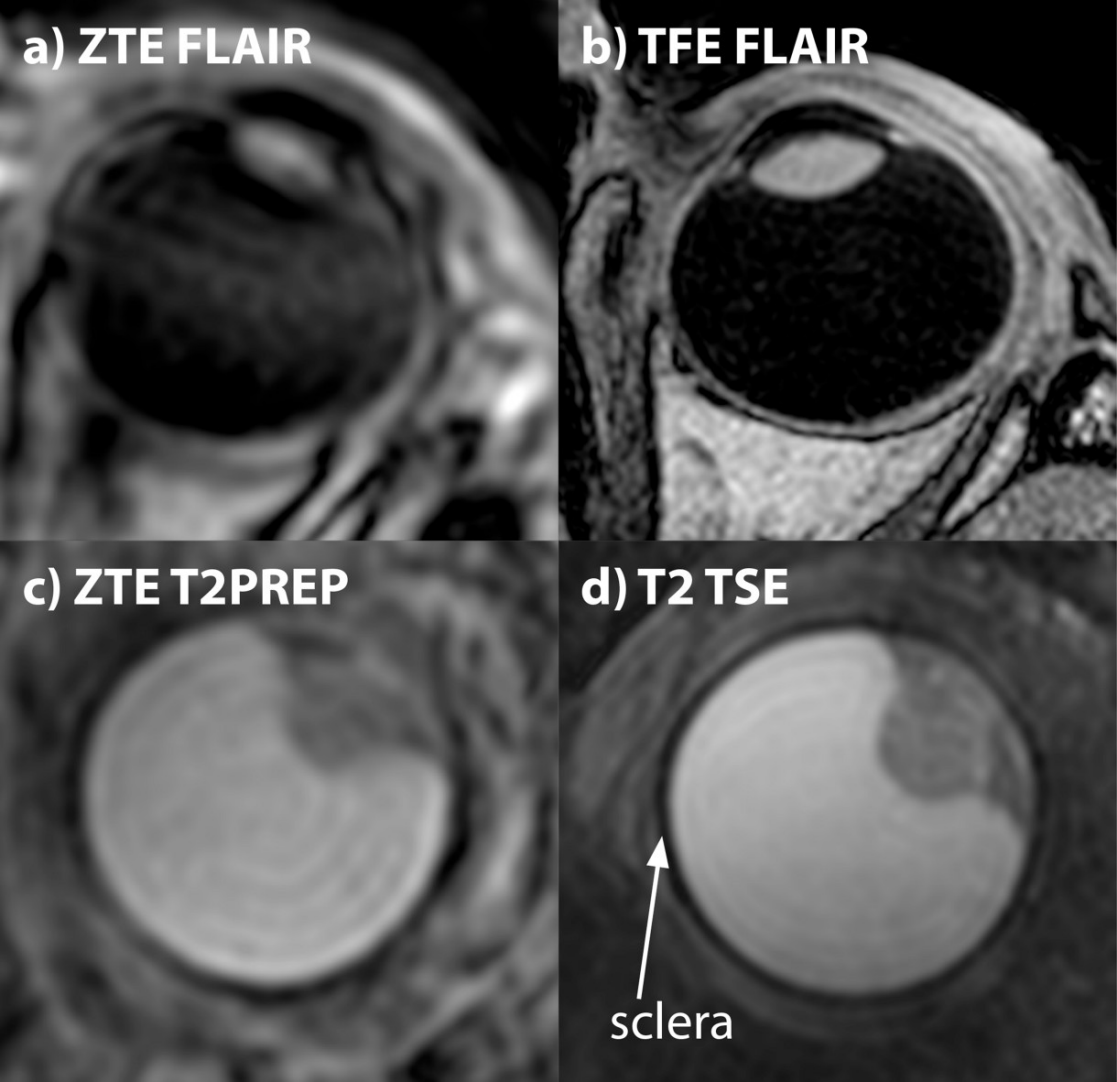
Comparison between magnetization prepared ZTE imaging and conventional gradient echo (TFE) Cartesian imaging. (a,b) Magnetization prepared FLAIR images of a healthy subject, show some contrast washout in the (1mm)^3^ ZTE scan, whereas complete suppression of the vitreous body is achieved in the Cartesian acquisition. (c,d) T_2_-prepared images of UM-patient 5. Although both acquisition methods result in a similar contrast, the higher resolution of the Cartesian imaging allows for a better delineation of the tumor-sclera interface compared to the (0.8mm)^3^ ZTE images.
